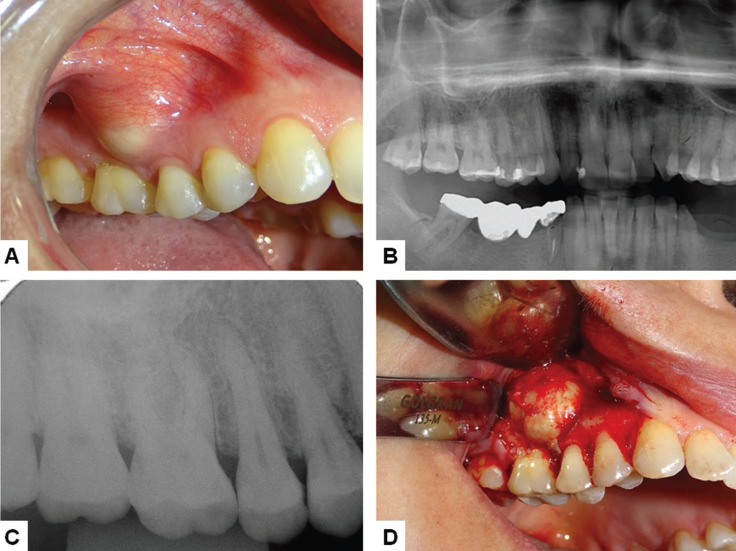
Case 1. A. Intraoral appearance of peripheral odontogenic keratocyst showing a well-defined nodular sessile swelling covered by normal oral mucosa in the upper right buccal gingiva between the second premolar and first molar. B. Panoramic radiograph showing no bone alterations in the right maxillary bone. C. Periapical radiograph showing no bone alterations in the area of the second premolar and first molar. D. Transoperative aspect showing a well-defined yellowish lesion superimposed to the cortical bone.
